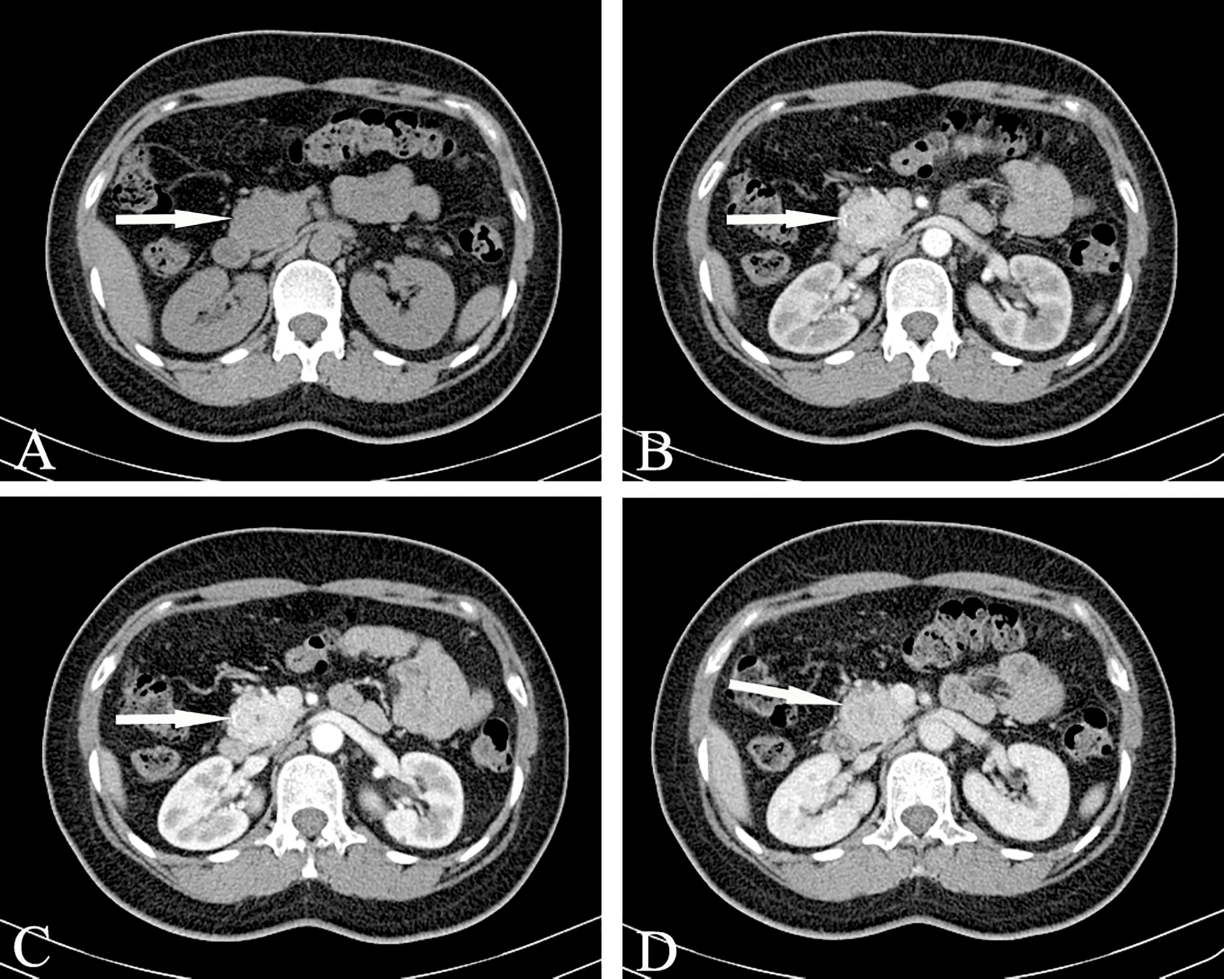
Solid serous cystadenomas, SSCA (Female, 43y, physical examination revealed a pancreatic mass for three weeks). (A–D) The CT plain scan, arterial phase, portal and venous phase at the same level; (A) The CT plain scan showed a solid mass in the head of the pancreas, with a CT value of about 31HU. (B) In the arterial phase, the mass of the pancreatic head was obviously enhanced, with a CT value of about 123HU. The focal low enhancement area can be seen in the center. (C) In the portal phase, the neoplasm showed progressive significantly enhancement, at this time, the CT value was 163HU; (D) The contrast wash-out can be seen in the venous phase of the mass, with a CT value of about 120HU. The overall manifestation was solid tumor with rich blood supply of pancreas. The white arrows in the Figures only indicate the location of the neoplasm.
